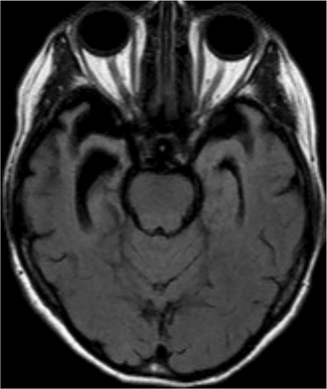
A 63-year-old female who presented with 2 years history of cognitive decline, mainly in semantic and visual recognition functions. On axial FLAIR image, there is asymmetric temporal lobe atrophy without gliosis, right greater than left. Based on cognitive tests, clinical presentation and imaging findings the diagnosis of semantic type of fronto-temporal dementia was made. This pattern of atrophy should be distinguished from post-traumatic encephalomalacia following bilateral temporal contusions, based on clinical history and the absence of gliosis and blood byproducts on imaging
